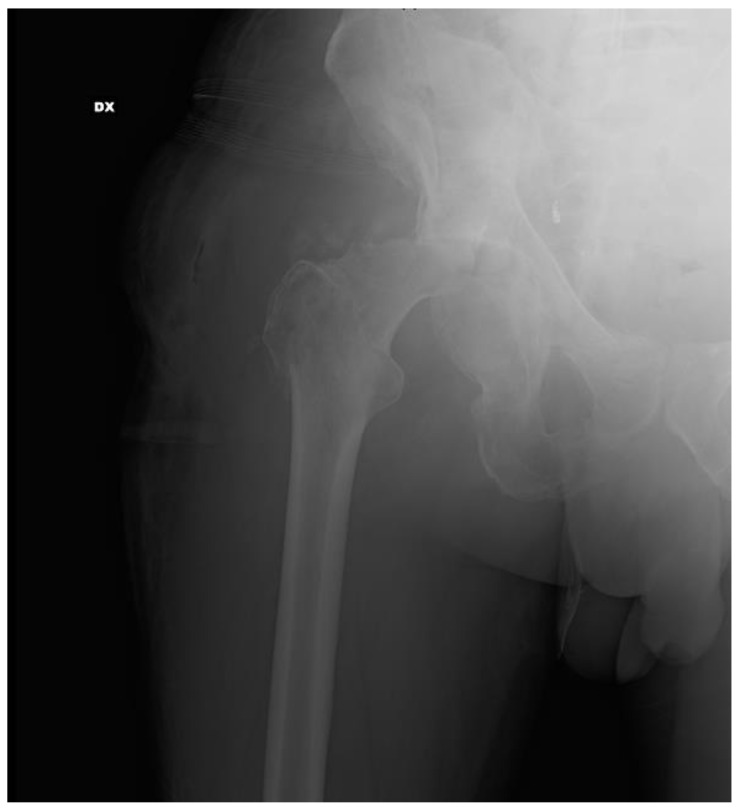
X-ray of the pelvis after the implant removal.
